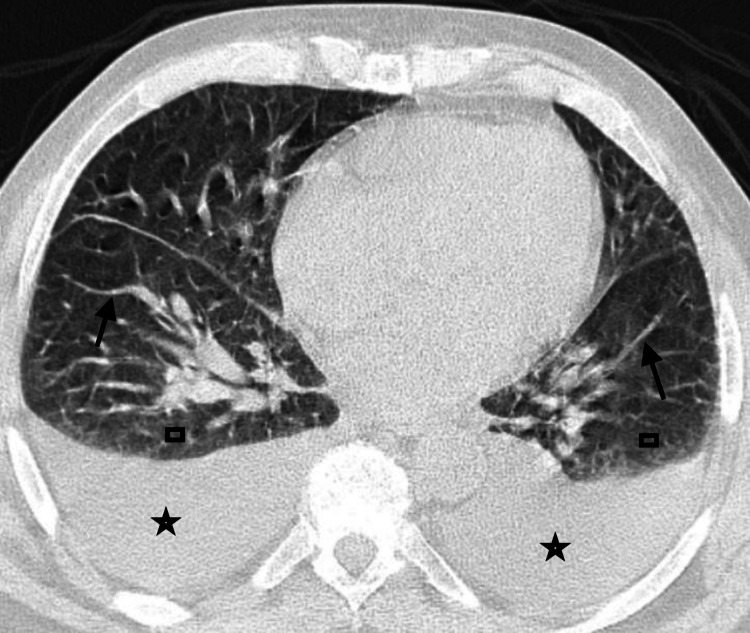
The chest CT scan shows bilateral pleural effusions (stars) with septal thickening (arrows) and patchy infiltrates (rectangles). CT: computed tomography
